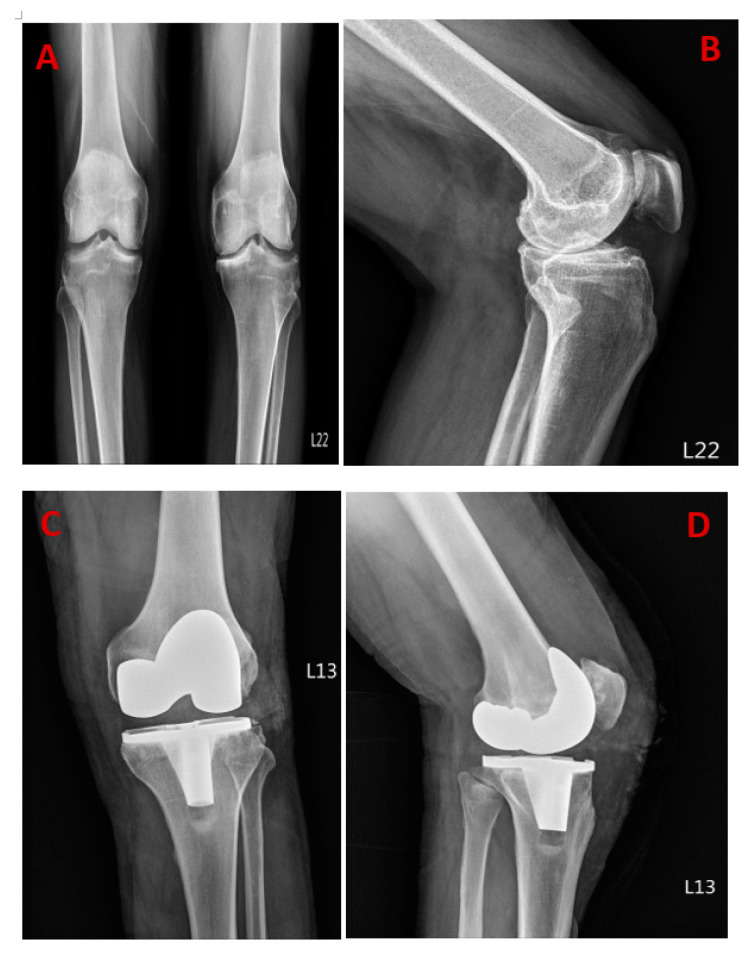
Initial radiography images before total knee replacement (A,B) with successful post-surgery radiography (C,D).
